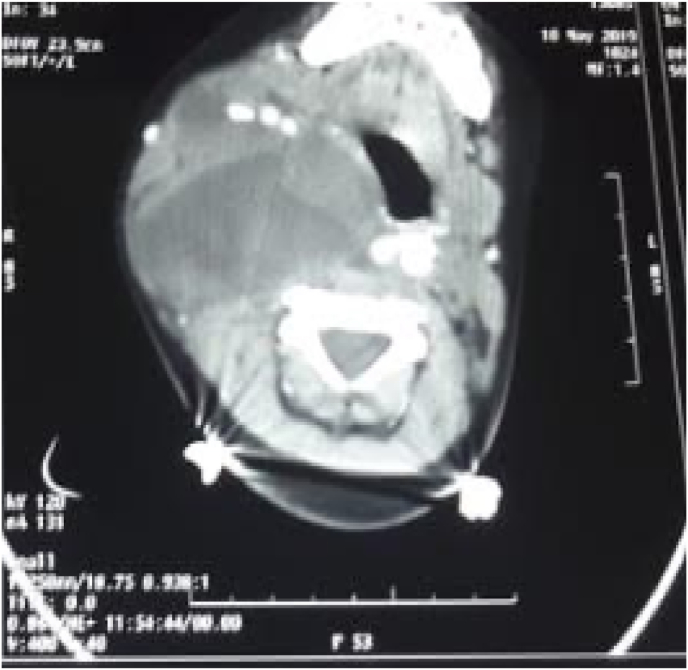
CT scan (C+)– axial section-hypodense mass compressing the aerodigestive tract.
